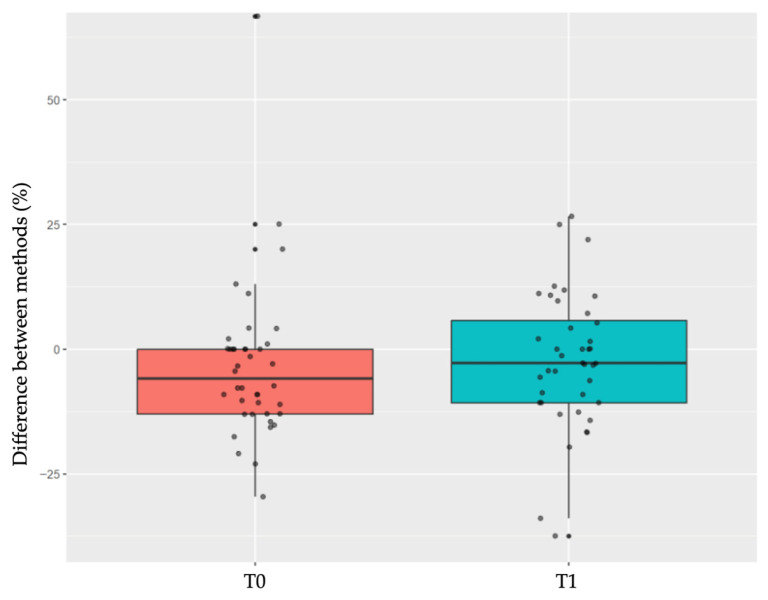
Distribution of relative differences between peri-implant soft tissue thickness measurements obtained using the PIROP ultrasound-based system and the reference clinical method at baseline (T0) and at the three-month follow-up after closed healing (T1). Boxplots represent the median and interquartile range, with individual paired measurements shown as points.
